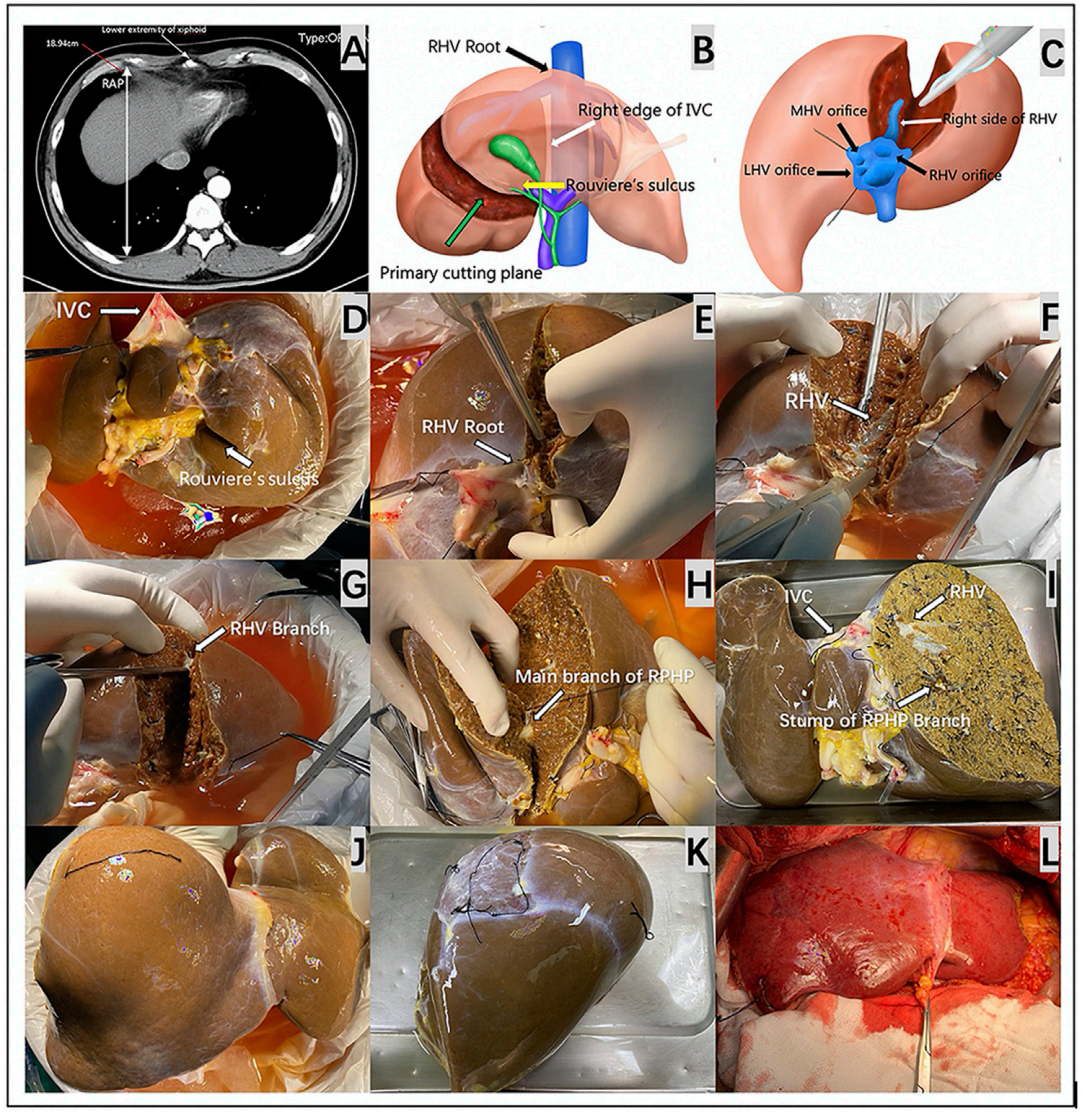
The key preoperative assessment and surgical procedures for HuaXi-eRPS. (A) The longest RAP vertical distance between the anterior and posterior parts of the ribs at the lower extremity of the xiphoid process is preoperatively measured on a CT scan for the recipient. (B) The primary cutting plane for HuaXi-eRPS is designed according to the right side of the RHV root (black arrow) entering into the suprahepatic IVC, right edge of the retrohepatic IVC (white arrow), and Rouviere’s sulcus (yellow arrow). (C) Parenchymal transection is designed to be started from the cranial side of the main RHV to the caudal direction, and the right side of the RHV (white arrow) is used as the surgical marker to navigate the intrahepatic transection. (D) The view on the visceral surface of the whole liver graft. IVC (long arrow); Rouviere’s sulcus (short arrow). (E) Parenchymal transection is started from the cranial side of the main RHV root (arrow) to the caudal direction. (F) The right side of the RHV (arrow) is used as the surgical marker to navigate the intrahepatic transection. (G) Dissection of the RHV branch (arrow) entering into segment VI. (H) Dissection of the main branch of RPHP (arrow). (I) The view on the visceral surface of the remnant liver graft after HuaXi-eRPS. (J) The view on the diaphragmatic surface of the remnant liver graft after HuaXi-eRPS. (K) The view on the diaphragmatic surface of the resected right posterior sector. (L) Implantation of the reduced-size liver graft into the recipient. HuaXi-eRPS, HuaXi-*ex vivo* right posterior sectionectomy; IVC, inferior vena cava; LHV, left hepatic vein; MHV, middle hepatic vein; RAP, right anteroposterior; RHV, right hepatic vein; RPHP, right posterior hepatic pedicle.
